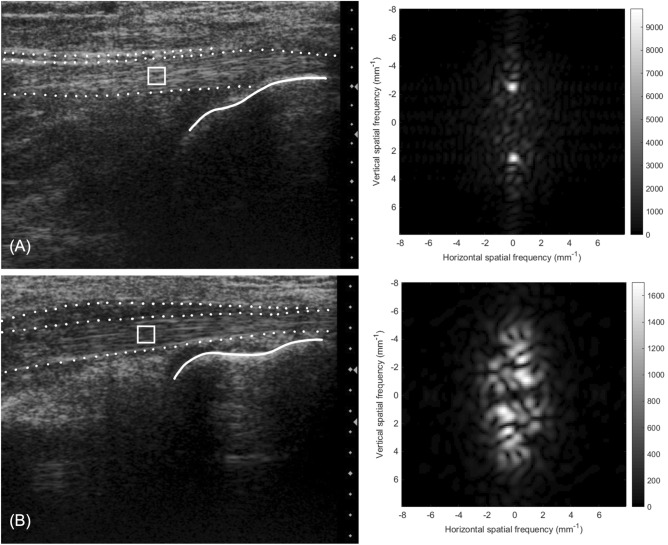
Frequency analysis of FHL tendon ultrasound images. Left: original B-mode image of FHL tendons with a sample kernel selection [white square, region of interest (ROI)] for a representative healthy dancer A and dancer with tendinopathy B. Dotted lines indicate outline of FHL tendon proper and paratenon. Right corresponding graphic representations of the frequency spectrum analysis of the selected ROI
